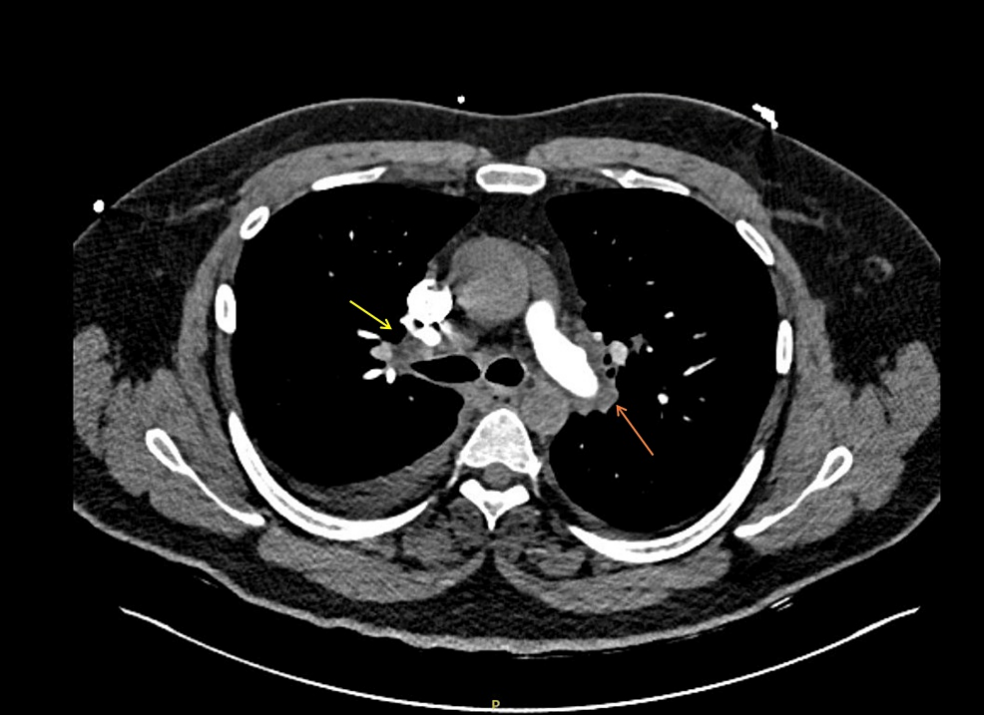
Computed tomography of the chest depicting enlarged mediastinal (yellow arrow) and hilar (orange arrow) lymph nodes.
